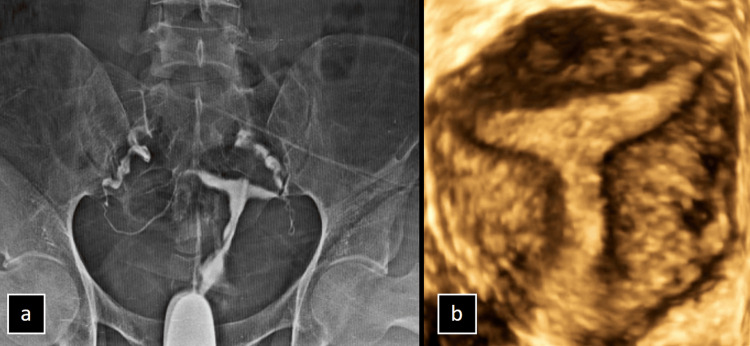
(a and b) Imaging of the T-Shaped Uterus in Patients With Infertility (a) Hysterosalpingography (HSG) image of a 35-year-old female with infertility reveals a small endometrial cavity with a T-shaped configuration, irregular and truncated bilateral fallopian tubes, and bilateral peritoneal spillage of contrast, suggestive of a "T-shaped" uterus. (b) 3D ultrasound image of a 31-year-old female revealing a “T-shaped" uterus.
